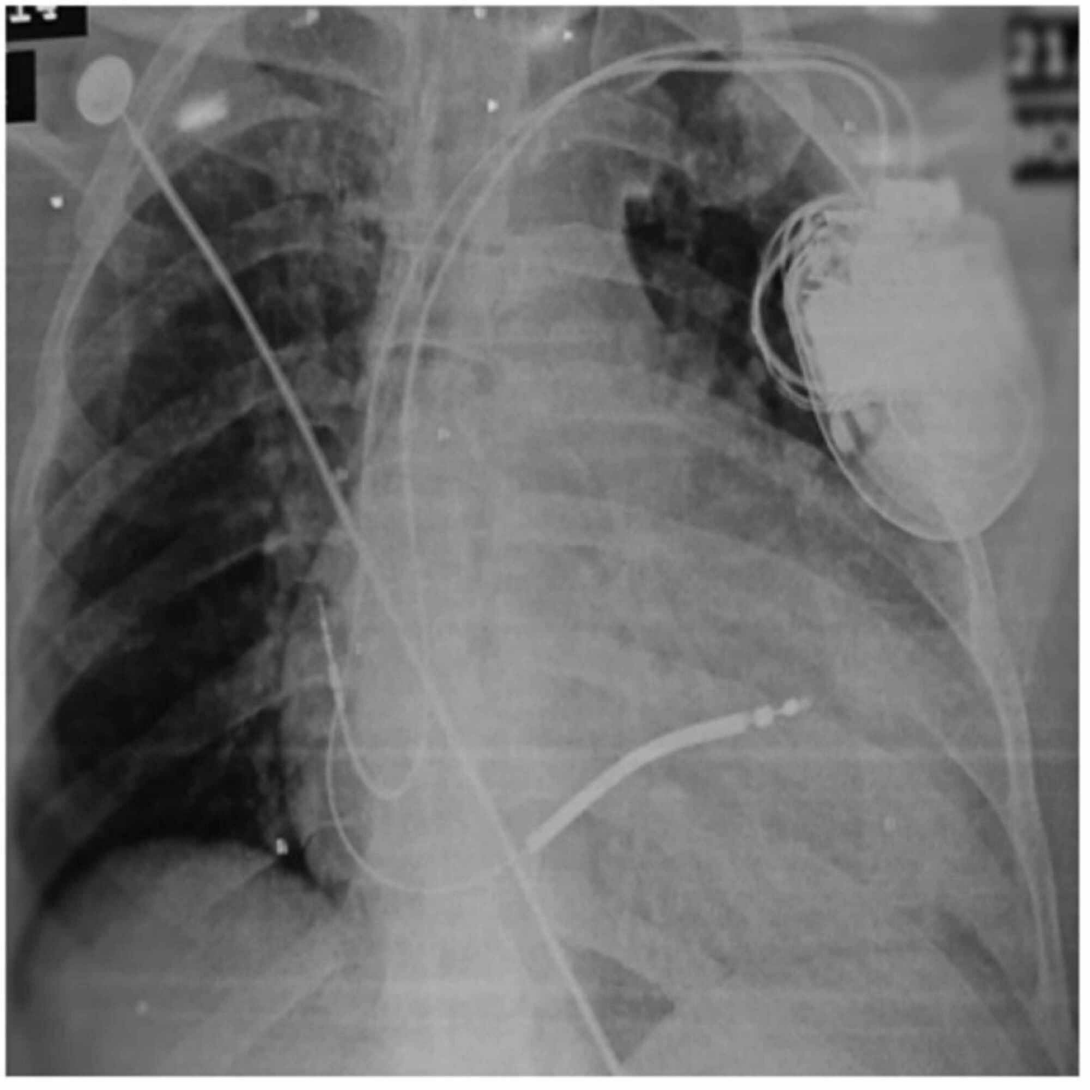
Post-ICD chest x-ray (AP-view) ICD: implantable cardiac defibrillator; AP: anteroposterior.
